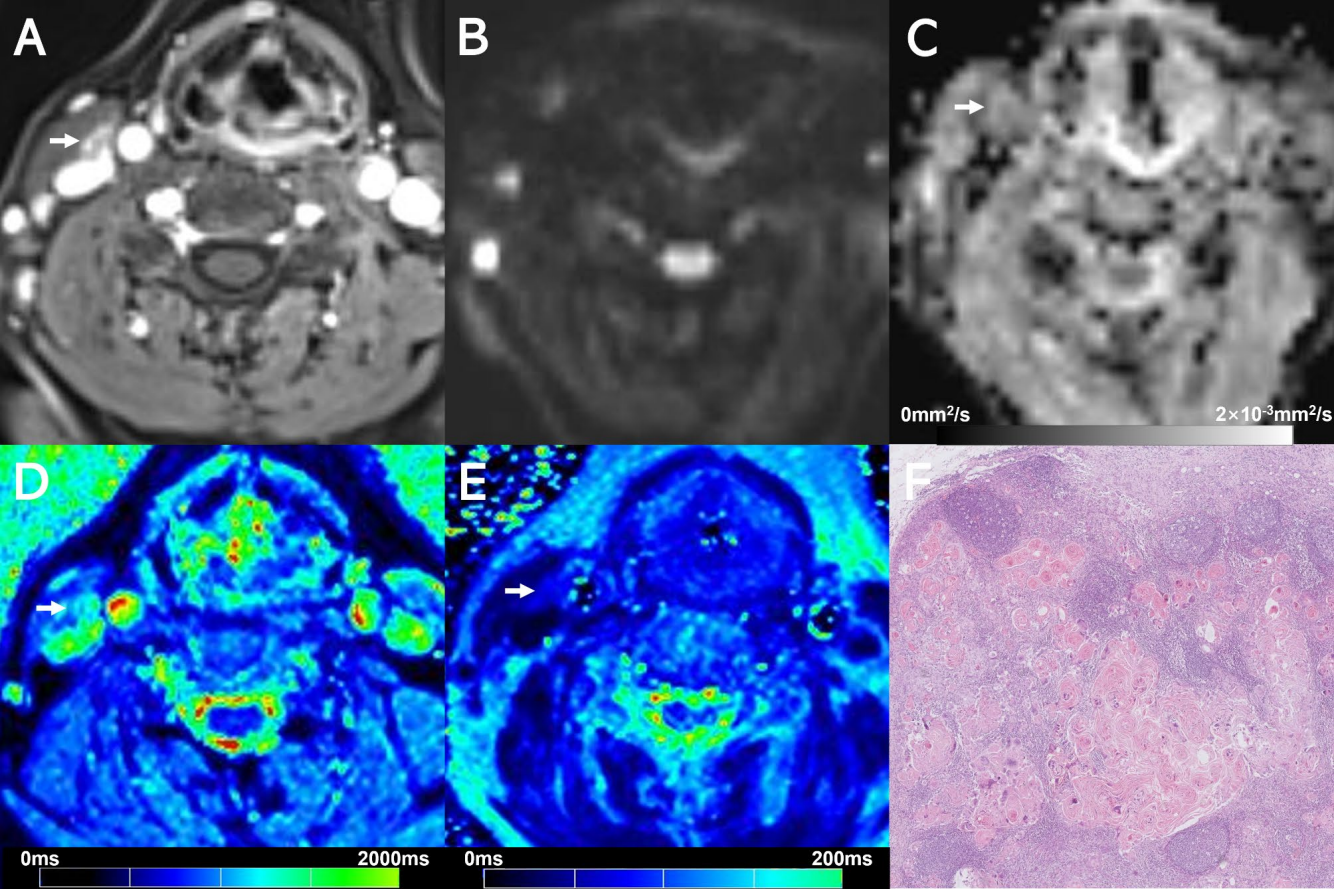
Images show a 5.0-mm size metastatic lymph node (arrows) at right level III from a 63-year-old female with oral cavity squamous cell carcinoma. (A) Contrast-enhanced T1-weighted image shows the lymph node (arrow). (B) DWI with a b-value of 800 mm^2^/s shows a hyperintense area. (C) The ADC map on the targeted lymph node shows a hypointense signal, an ADC value of (1.003 × 10^− 3^mm^2^/s). (D) T1 mapping and (E) T2 mapping show a T1 value of 1434.3 ± 148.8 ms and a T2 value of 64.4 ± 4.1 ms. (F) Section through the same lymph node showing metastatic deposit
